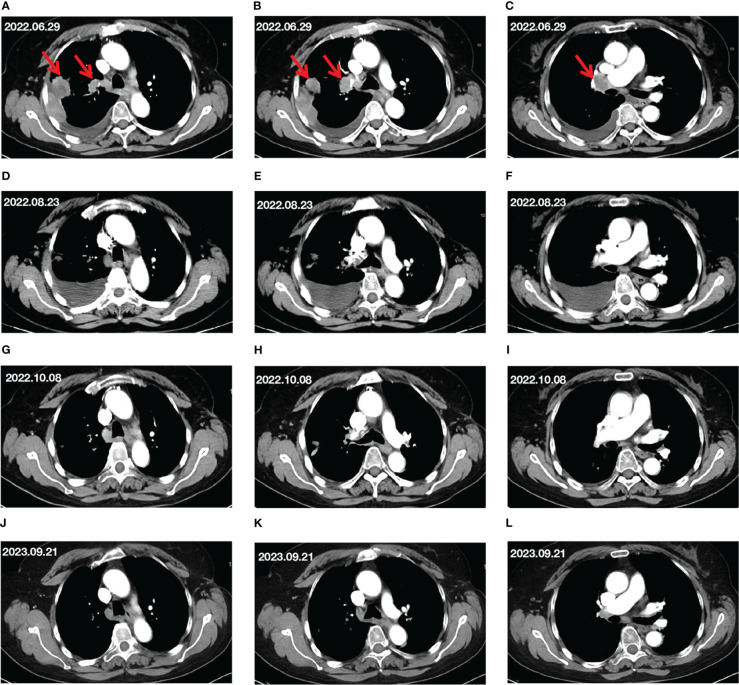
Changes in target lesion during treatment. (A–C) CT scan before treatment. (D–F) CT scan after 2 cycles of treatment. (G–I) CT scan after 4 cycles of treatment. (J–L) CT scan after 15 months of treatment. The red arrows mark the target lesions.
